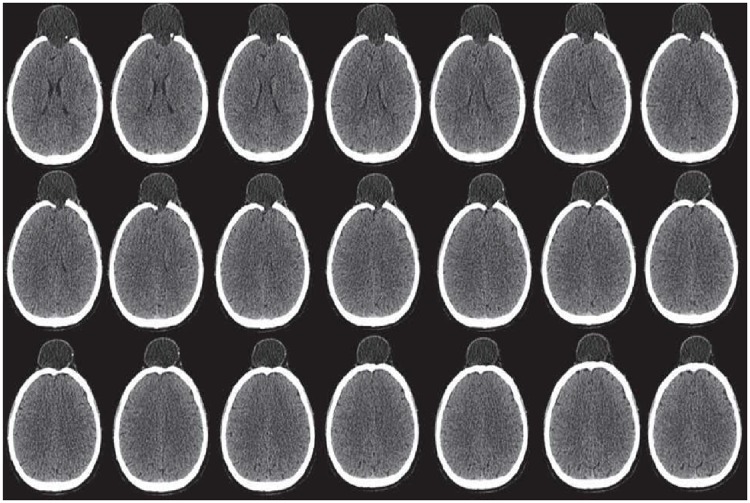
CT scanned images of patient with head injury.
